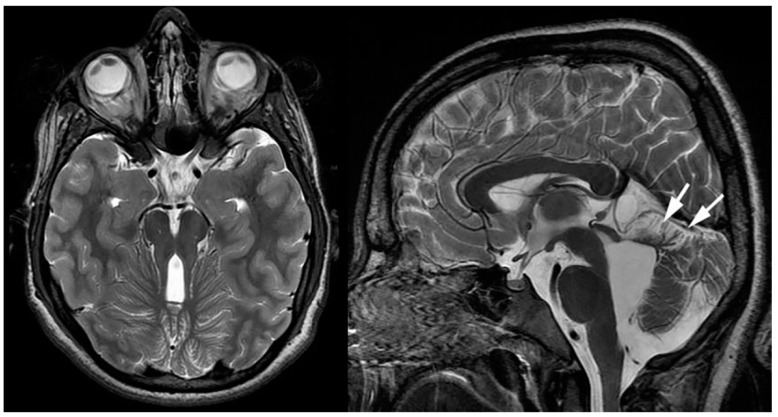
Axial and sagittal T2-weighted MRIs of a teenage patient with confirmed Joubert-Boltshauser syndrome show the characteristic molar tooth appearance of the brainstem on axial imaging with thickened and horizontal-coursing cerebellar peduncles and a nearly completely absent vermis. A small malformed/hypoplastic vermis is noted on the sagittal imaging (white arrows).
